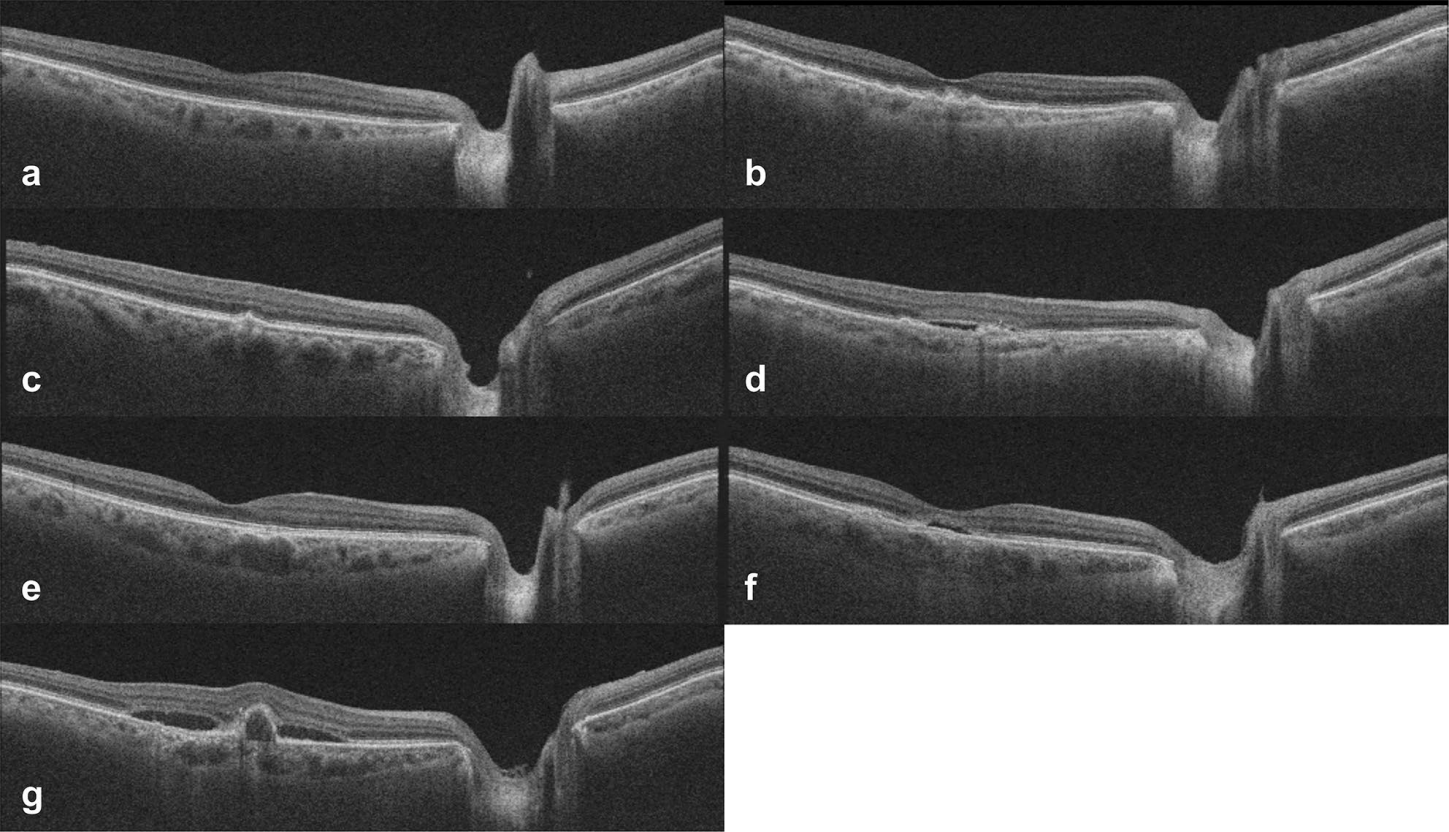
Representative swept-source optical coherence tomography images of eyes with (a) normal control, (b) early age-related macular degeneration (AMD) with soft drusen or reticular pseudodrusen, (c) pachydrusen, (d) classic exudative AMD, (e) pachychoroid pigment epitheliopathy, (f) pachychoroid neovasculopathy, and (g) polypoidal choroidal vasculopathy. The subfoveal choroidal thicknesses (CT), peripapillary CTs and the ratios of subfoveal CT to peripapillary CT are (a) 287 μm, 117 μm, 2.45, (b) 142 μm, 102 μm, 1.39, (c) 322 μm, 141 μm, 2.28, (d) 143 μm, 78 μm, 1.83, (e) 337 μm, 154 μm, 2.19, (f) 347 μm, 188 μm, 1.85, and (g) 264 μm, 132 μm, 2.00, respectively.
